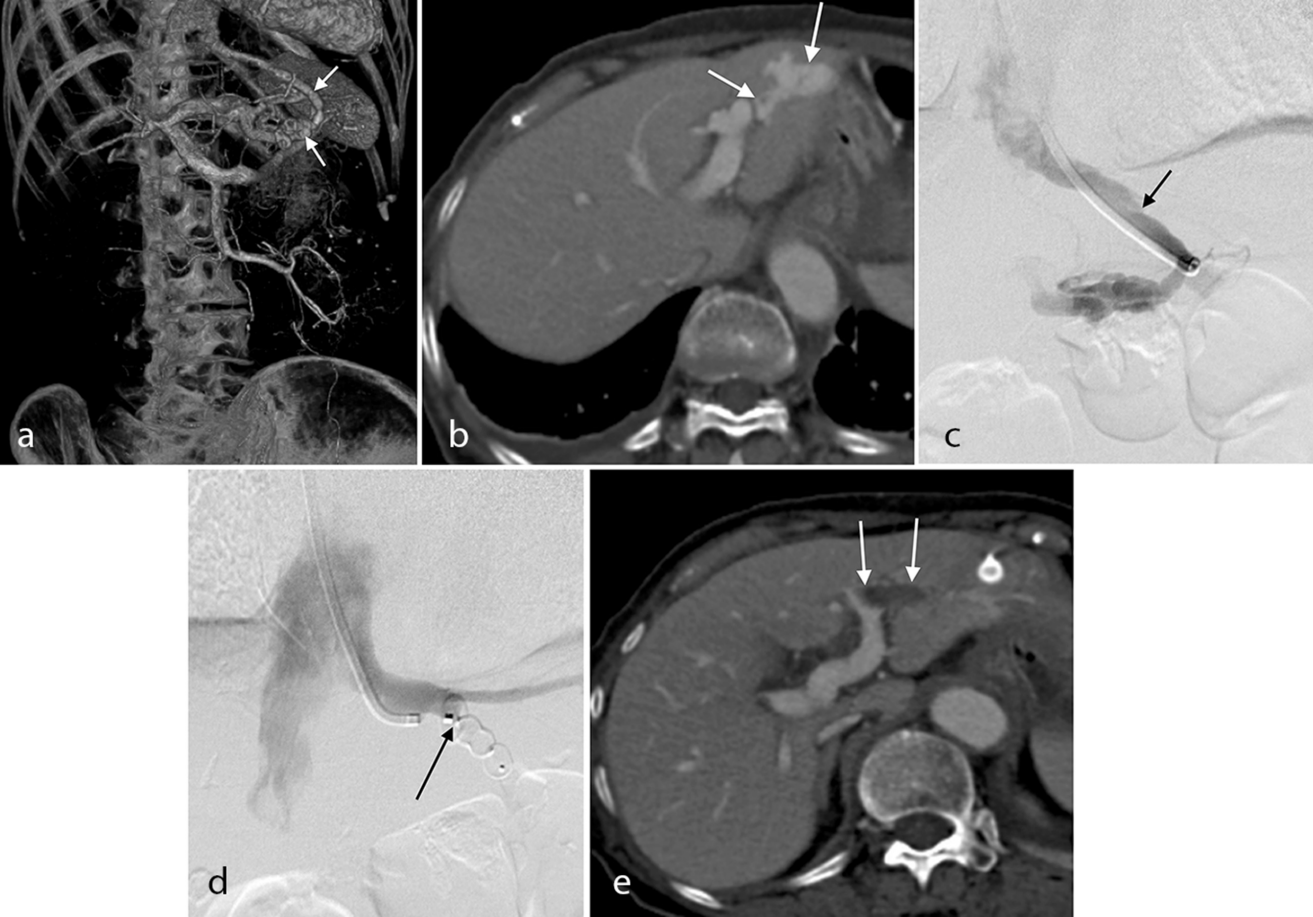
A 75-year-old female: (a, b) Pretreatment dynamic CT and volume rendering image based on dynamic CT shows a tortuous shunt between the portal vein (P3) and the left hepatic vein (arrows). (c) Venography after catheterisation of the left hepatic vein. The diameter of the left hepatic vein is 7 mm (arrow). (d) Venography after deploying the AMPLATZER Vascular Plug II: embolisation of the left hepatic vein is shown, and embolisation of the right hepatic vein is indicated (arrow). (e) Dynamic CT 1 month after the procedure shows complete embolisation of the portal vein hepatic vein shunt (arrows).
